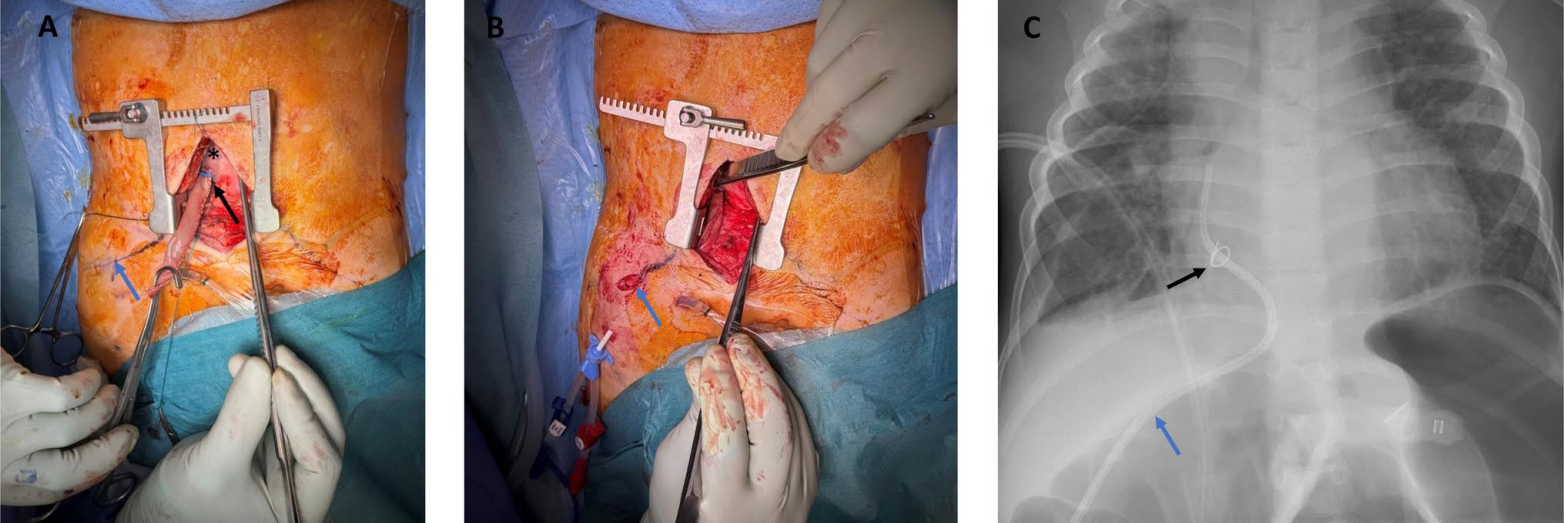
A, B Intraoperative image showing the pericardial tube with the radiopaque marker (black arrow), the right atrium (*), and the access sites: the lower mini-sternotomy and the subcostal incision (blue arrow). C Chest X-ray image showing the dialysis catheter with the radiopaque marker (black arrow)
